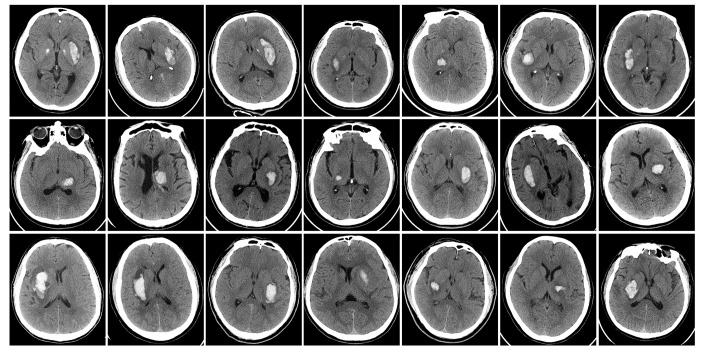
Baseline head CT of ICH patients immediately after admitting hospital.
